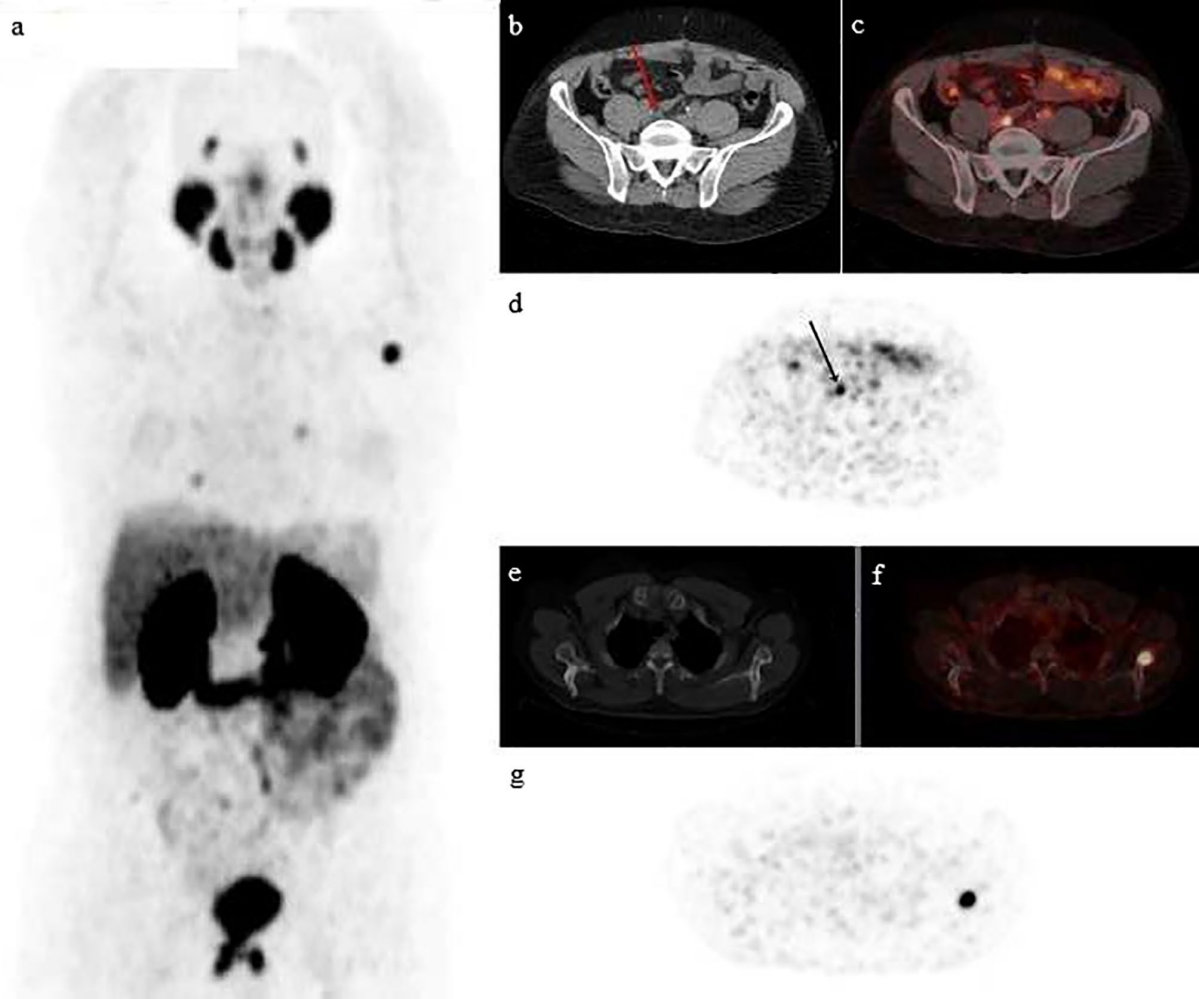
A 68-year-old patient status post radical prostatectomy. PSA level at time of acquisition: 9 ng/mL. Whole body 68 Ga-PSMAPET/CT was performed. Maximal intensity projection image (a) showing metastatic disease. Axial CT (b) showing disease in the right common iliac lymph node (red arrow). PET/CT (c) showing focal areas of radiotracer uptake in the apical region of the prostatic bed associated with pre-sacral radiotracer avid lymph node as well as multiple radiotracer avid bone lesions. PET image (d) shows focal uptake at the right common iliac node (black arrow). Axial thoracic CT (e) fused with PET (f) shows focal uptake in the left scapula. PET image (g) demonstrates this lesion.
